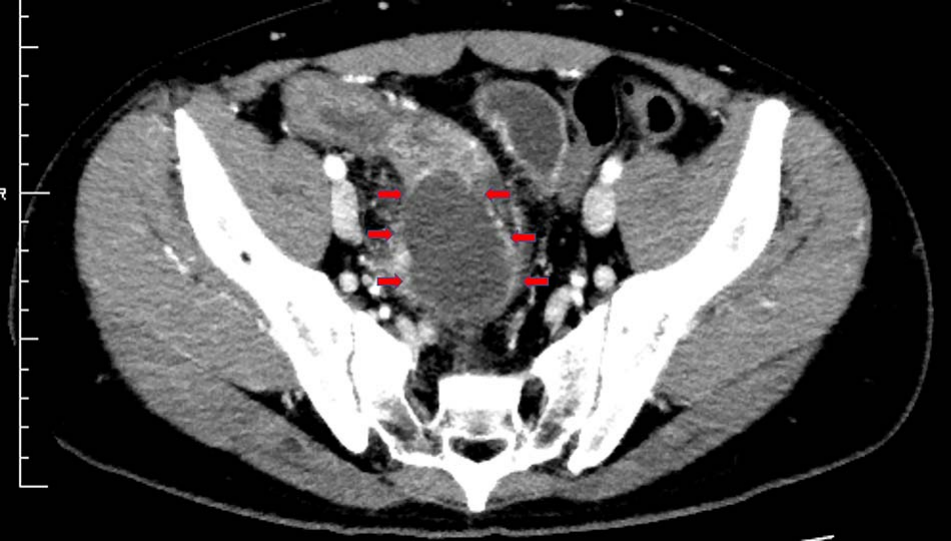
A contrast-enhanced computed tomography (CECT) scan showing a typical abscess finding of a 50-mm well-defined hypodense lesion with peripheral ring enhancing located next to the inflamed ileum (red arrows).
